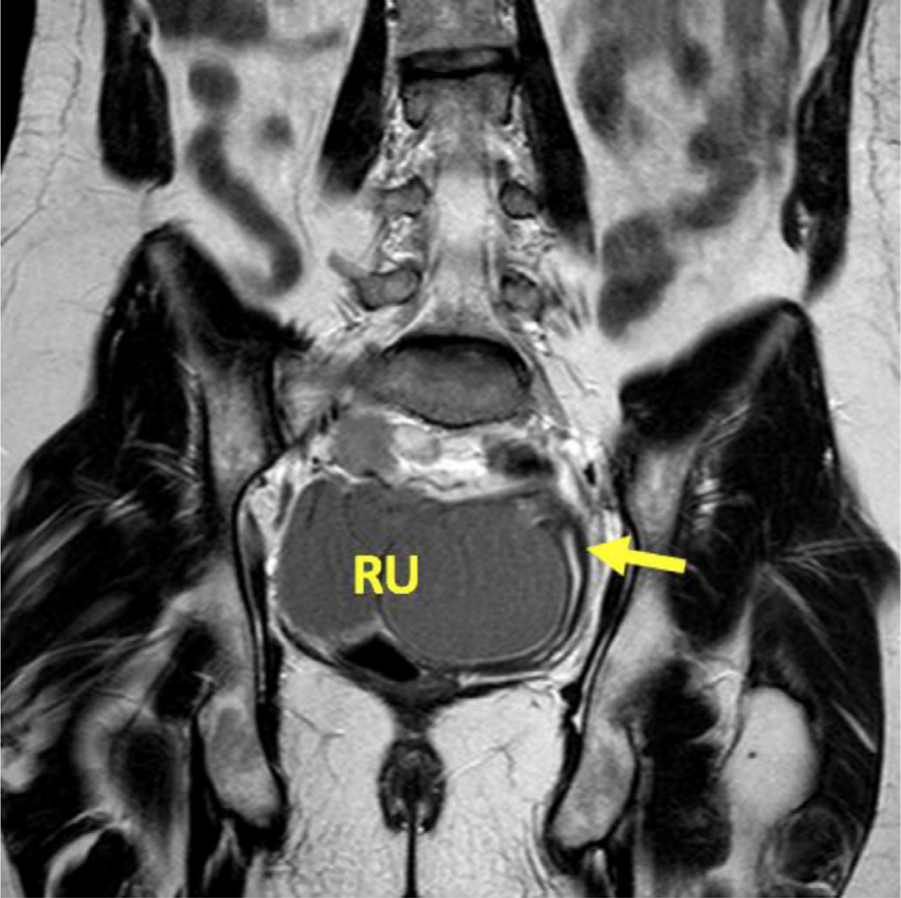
MRI T2-Weighted coronal image. Distended right uterus with blood products (RU) and collapsed left hemivagina (arrow).
